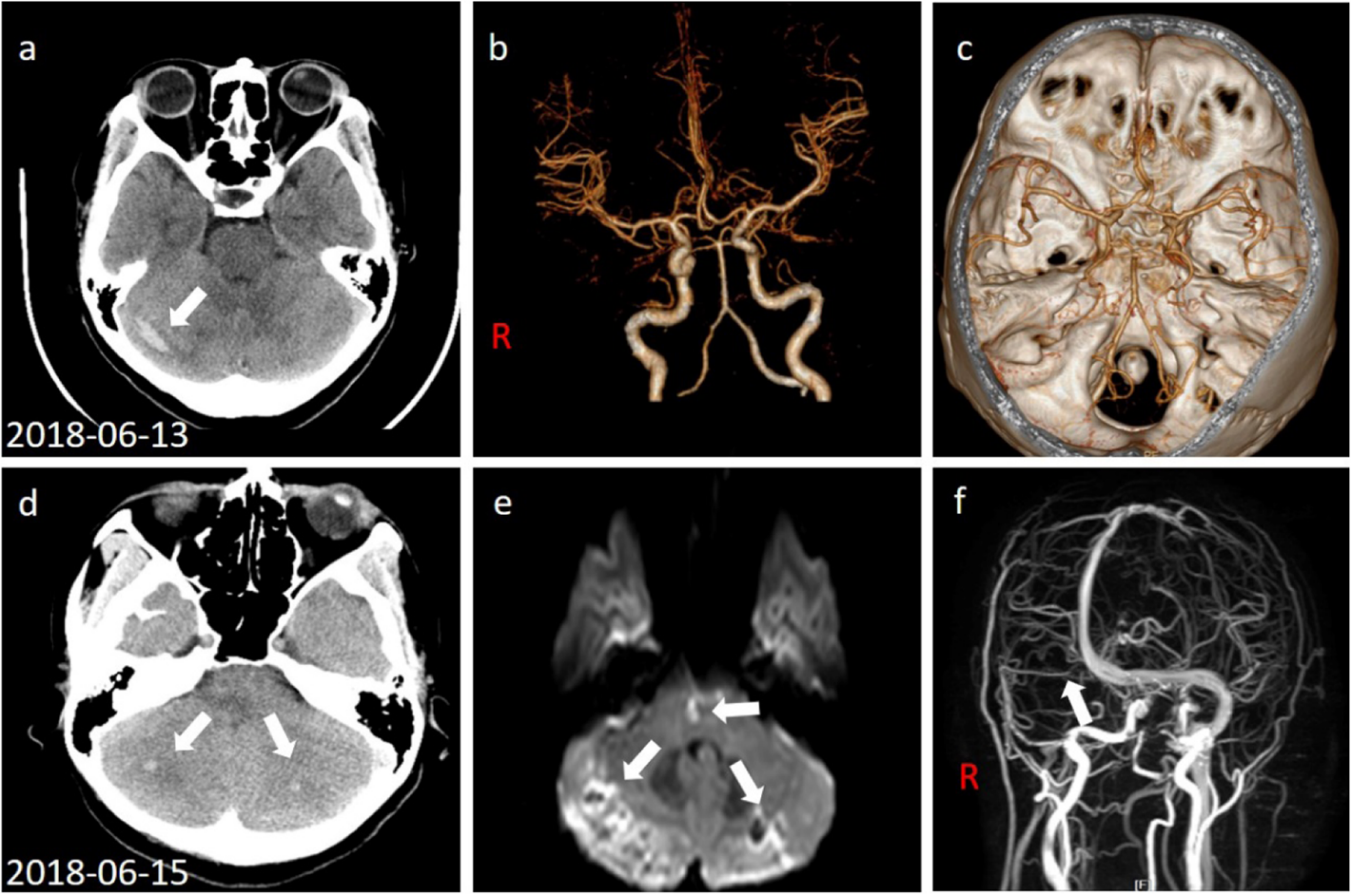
First CT and CTA scans on 2018-06-13, showed hyperdense region (white arrow) on the right cerebellum without arterial malformation (b, c). A second CT scan after the deterioration of clinical condition showed bilateral hemorrhage (white arrows) in the cerebella (d). Brain MRI indicated that acute lacunar infarction in pons (white arrow) with restricted diffusion on DWI (e). MRV indicated that the right transverse and sigmoid sinuses were thin and tortuous (f, white arrow)
